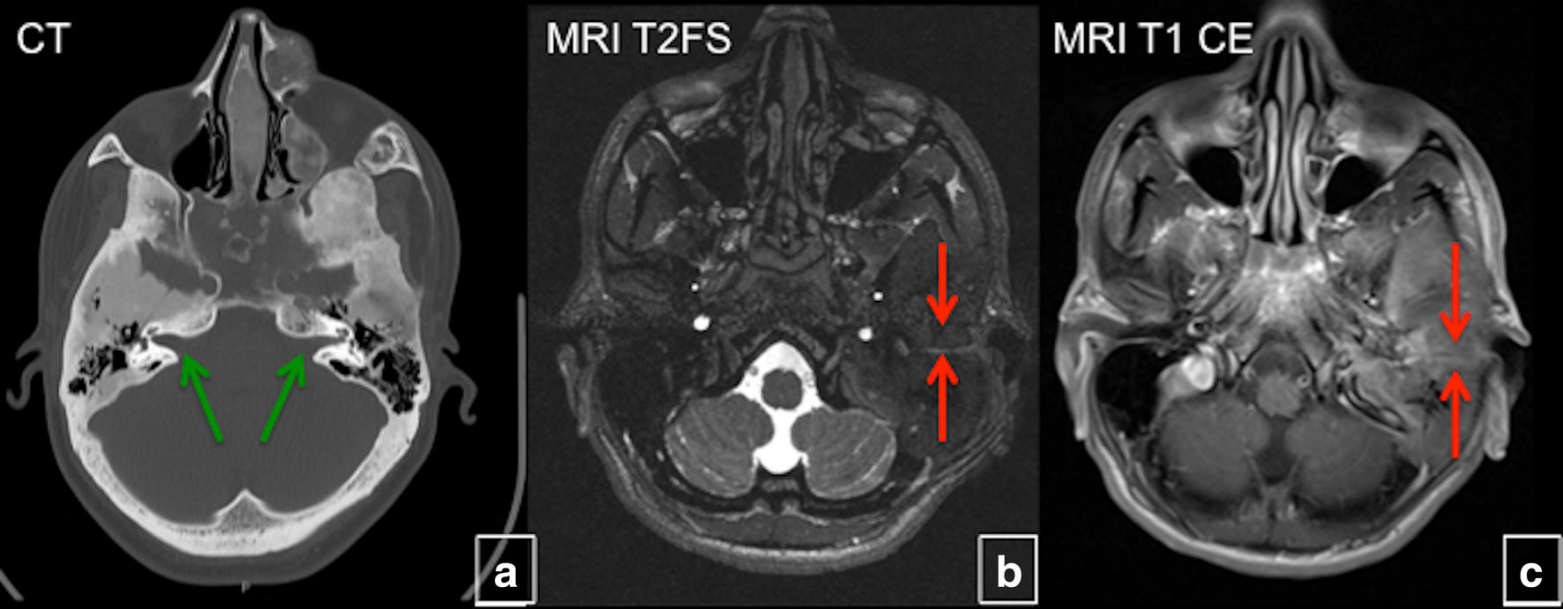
Evaluation of hearing loss in craniofacial fibrous dysplasia (FD). a Extensive fibrous dysplasia (FD) involving petrous bones bilaterally; however, the internal auditory canals are patent (*green arrows*). T2 fat-saturated (b) and T1 contrast-enhanced (c) MRI demonstrate a large FD lesion centred in the left petrous temporal bone with near complete obliteration of left external auditory canal (*blue arrow*), which contributes to patient’s hearing loss (*green arrow*)
